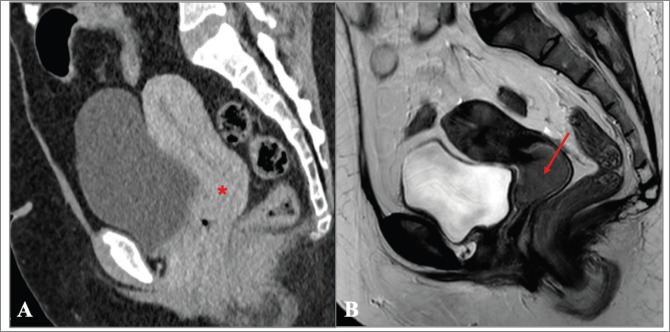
(a and b): Comparison between the CT performed in CV and MRI obtained in IPOLFG. (a): On the sagittal plane CT of the pelvis obtained in the venous phase, the cervix appeared normal (*) and no cervical tumour could be accurately depicted. (b): On the T2-weighted MR image in the sagittal plane performed 3 months later, the cervical tumour was clearly seen, measuring 3.5 cm of greatest axis (FIGO stage IB2).
